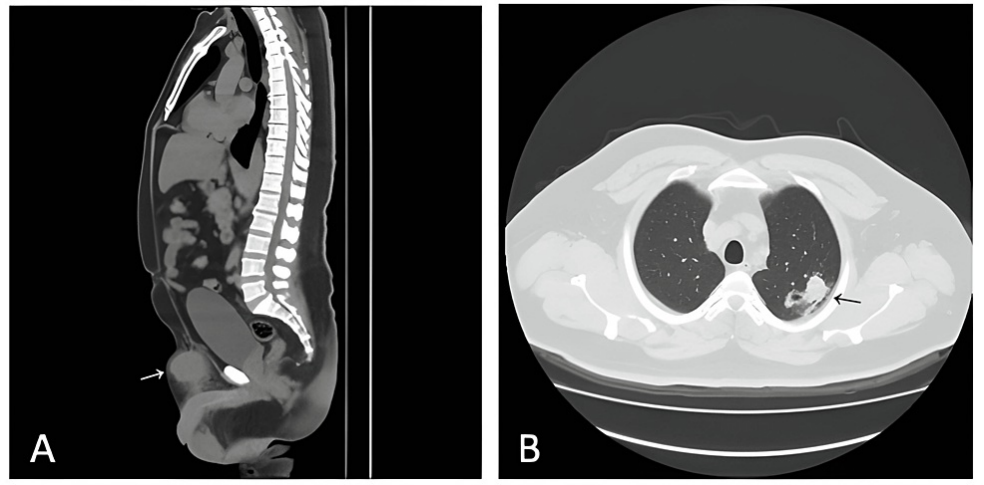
(A) Abdominopelvic CT scan with contrast, axial section: hypervascularized mass (white arrow) in subcutaneous cellular tissue in the pubic and suprapubic region suggestive of a neoplasm. (B) Chest CT scan with contrast, sagittal section: image (black arrow) suggestive of metastasis in the left lung parenchyma.
